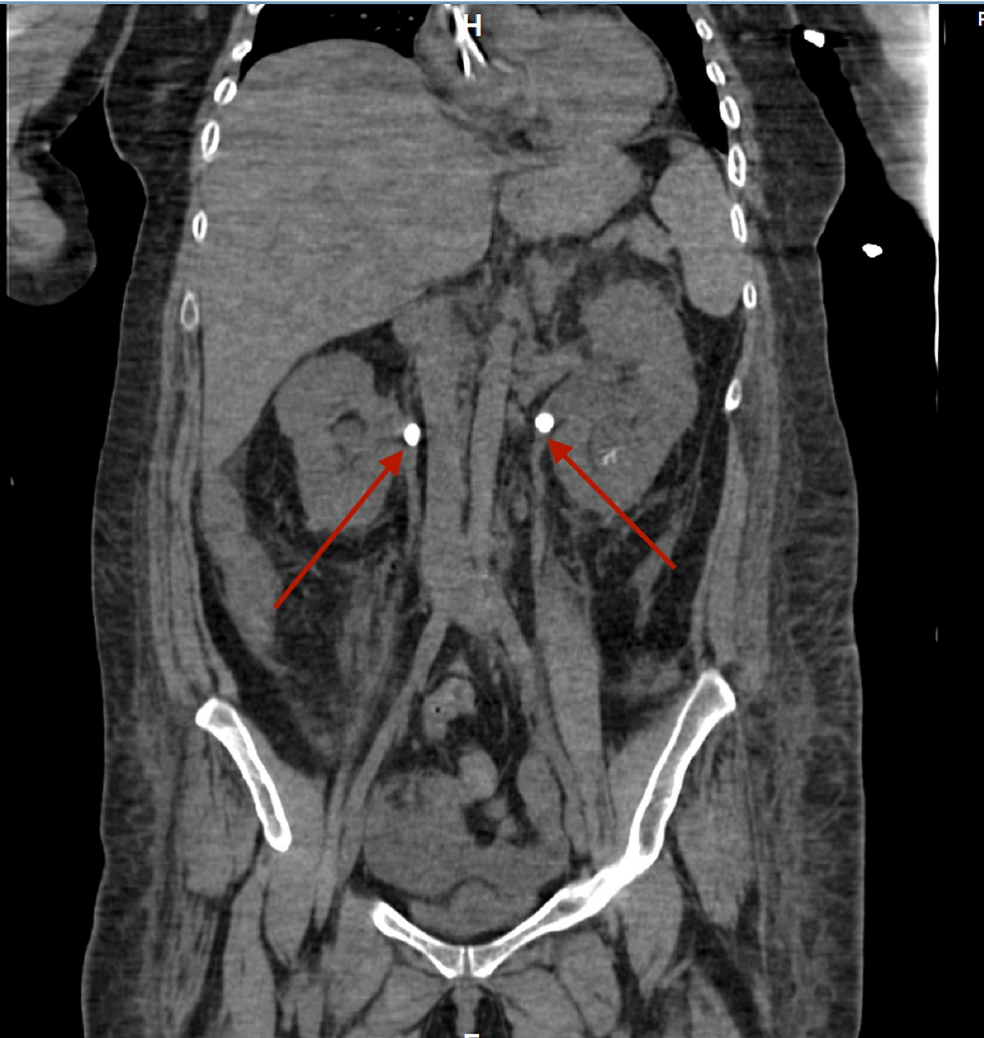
CT scan of the abdomen and pelvis showing bilateral obstructing stones at the ureteropelvic junction.
